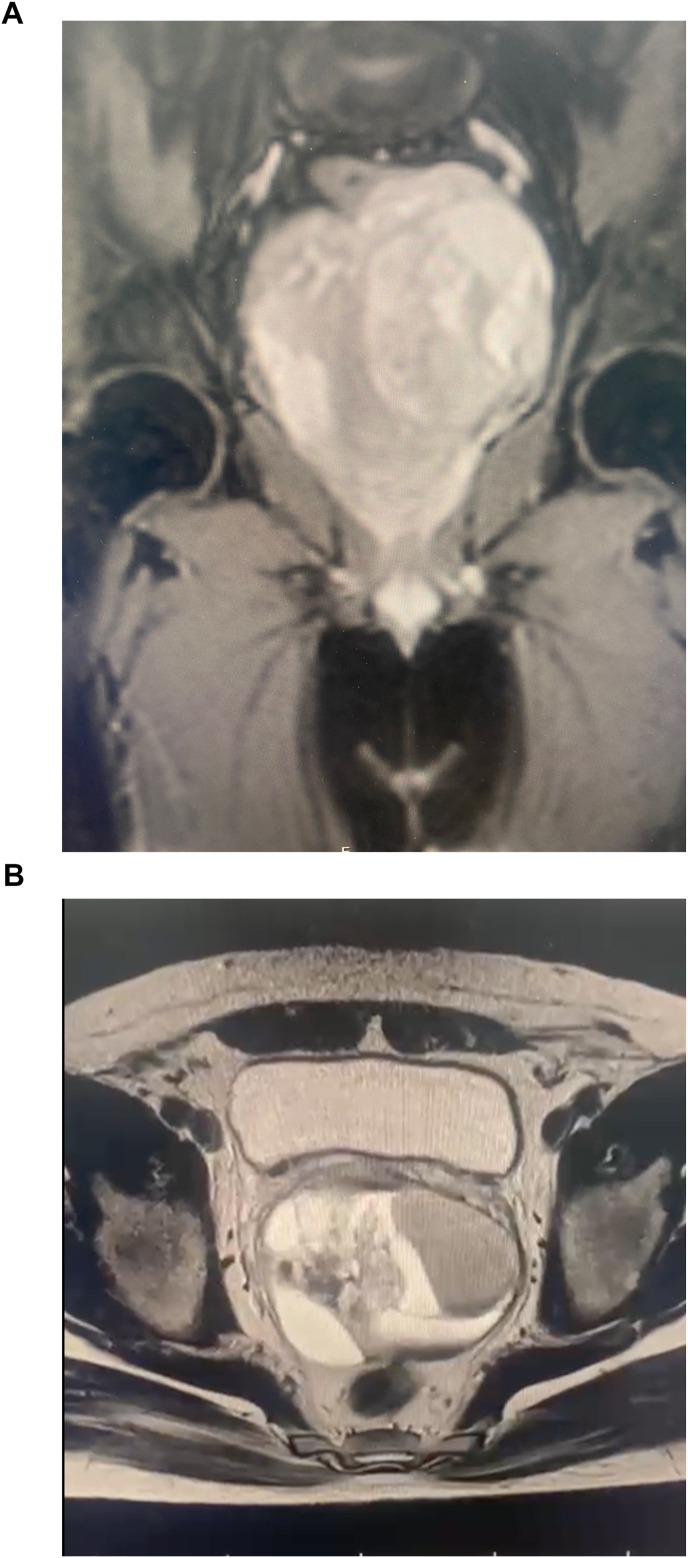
Pelvic MRI of the multilocular cystic mass. a Coronal T2-weighted image showing a large multilocular lesion located posterosuperior to the prostate, causing displacement of the rectum and bladder. b Axial T2-weighted image demonstrating heterogeneous signal intensity with internal septations and compression of adjacent pelvic organs.
